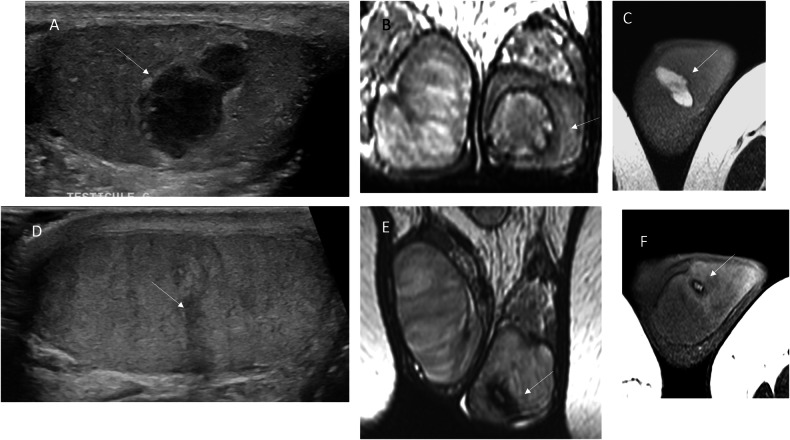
Spontaneous hematoma in a 38-year-old patient. Initial US and MRI were performed 4 days after symptom onset, with 6-week follow-up imaging. A (US), B (MRI, T2-weighted sequence) and C (MRI, T1-weighted sequence): Initial examinations showing features similar to other cases, with additional striated heterogeneous signal of the surrounding parenchyma. D (US) Follow-up US showing regression of the hematoma, leaving a small hypoechoic scar (arrow). E (MRI, T2-weighted sequence) and F (MRI, T1-weighted sequence): residual lesion with a marked hypointense rim consistent with hemosiderin deposition
